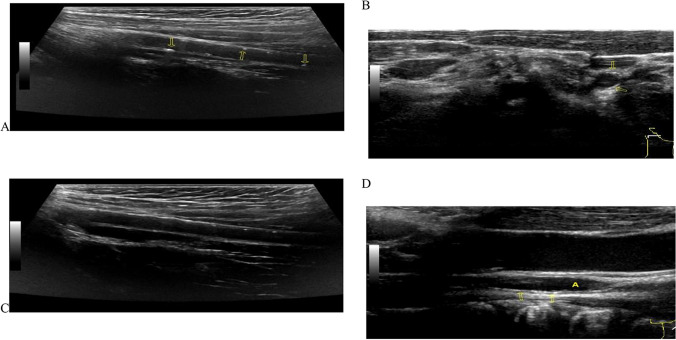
B-ultrasound images of the carotid and femoral arteries. The wall of the carotid and femoral arteries were thickened with multiple mottled calcifications. A Right femoral artery; B right carotid artery; C Left femoral artery; D Left carotid artery
